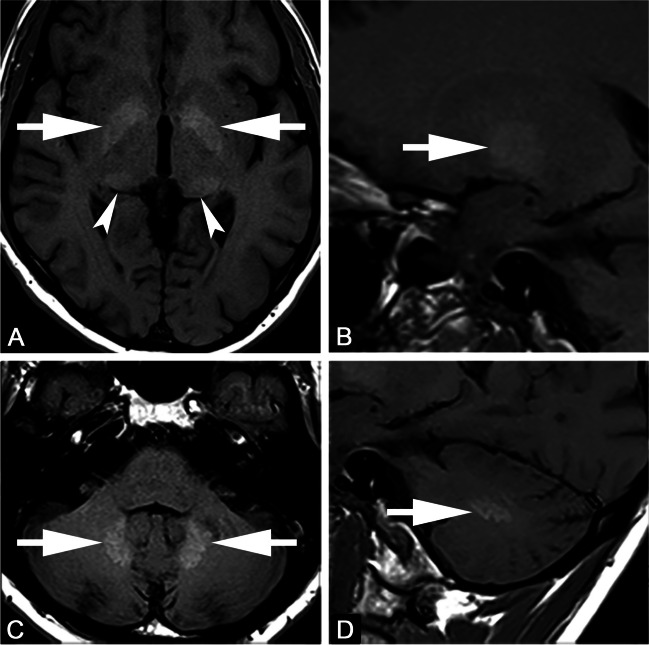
Axial (A, C) and sagittal (B, D) T1-weighted images. Forty-seven-year-old male with multiple sclerosis previously exposed to 14 intra-venous injections of gadodiamide. Globus pallidus and dentate nucleus hyperintensity on unenhanced T1-weighted images due to gadolinium deposition (arrows in A–D). Faint T1 hyperintensity of the pulvinar thalami is also seen (arrowheads in A)
